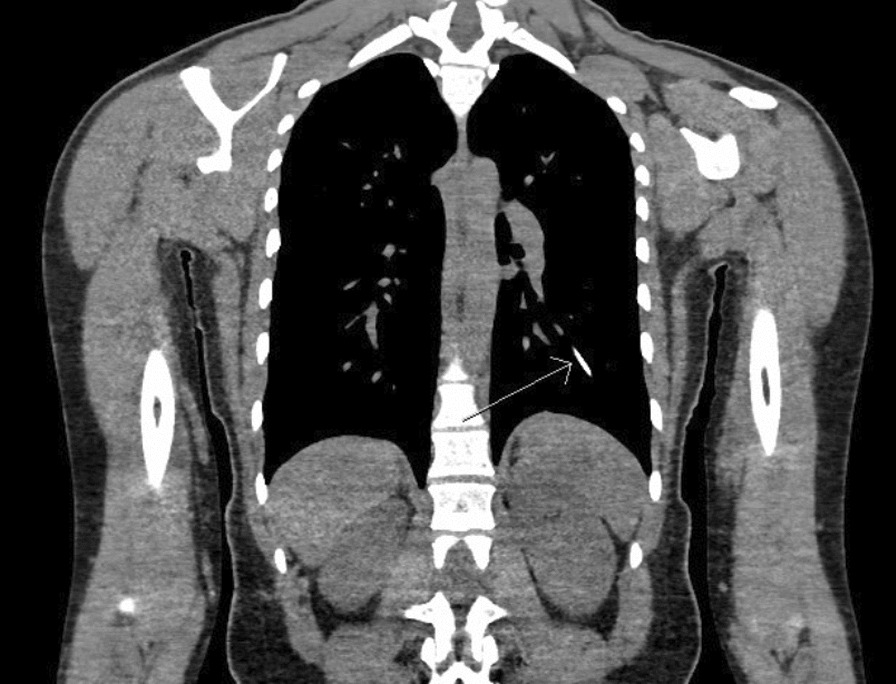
CT chest showing embolized Nexplanon (thin white arrow)
